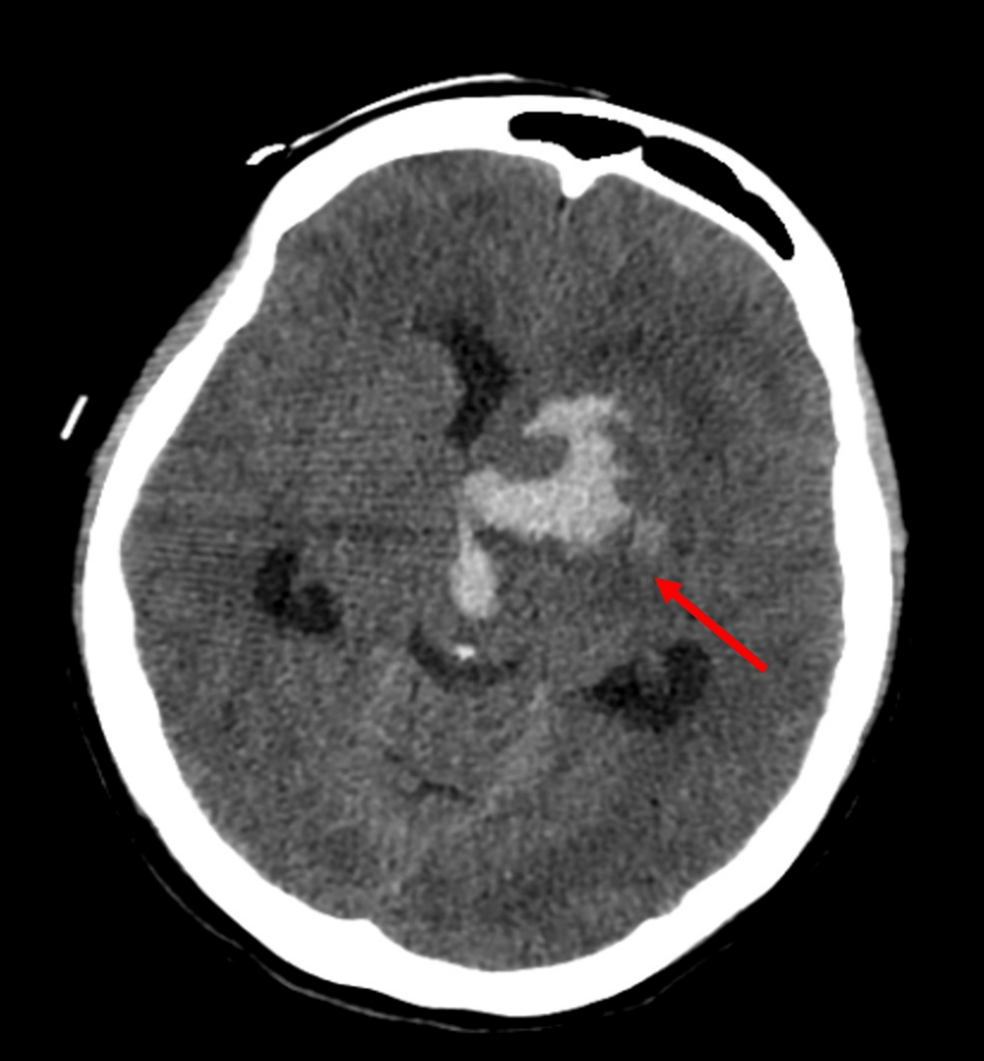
Brain CT Haemorrhagic lesion (arrow) in the left lenticulocapsular region and tetraventricular haemorrhage with ectasia of the left ventricular system and moderate hydrocephalus
